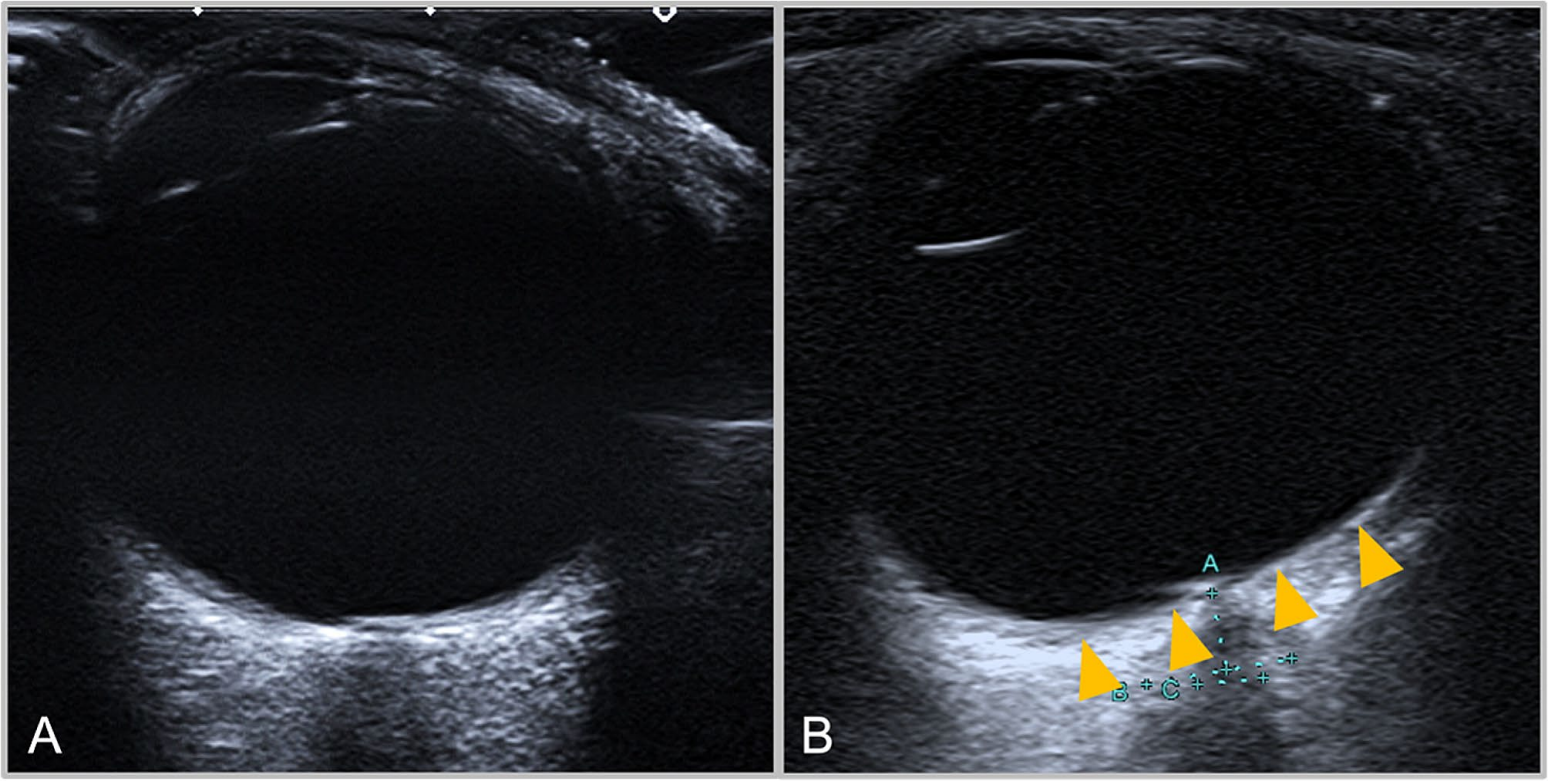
Two B-mode transorbital sonographic images of two individual globes in the axial plane. Image A shows a normal globe. Image B depicts posterior globe flattening in a globe with straightening of the outward convexity of the sclera at the area of attachment to the optic nerve (yellow arrowheads)
